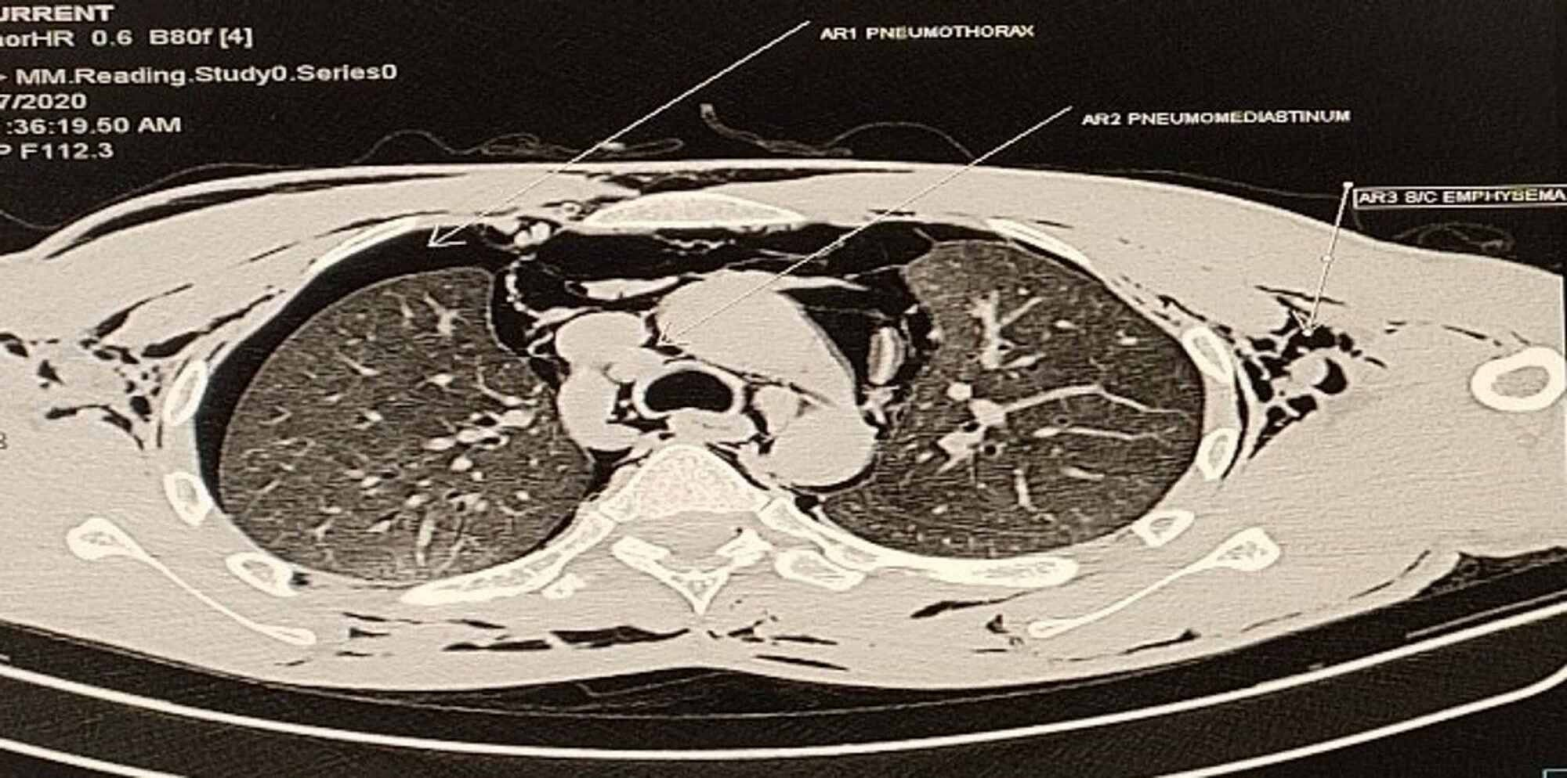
CT thorax showing pneumothorax, pneumomediastinum, and subcutaneous emphysema CT: computed tomography
